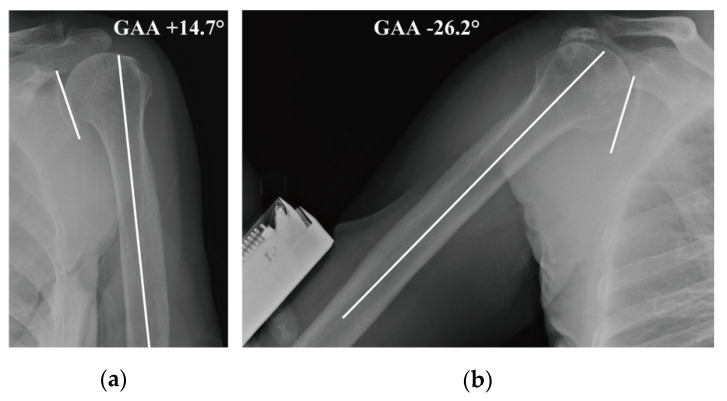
Radiographic glenohumeral adduction angle (a) Glenohumeral adduction angle (GAA) in a normal subject (b) GAA in a patient with rotator cuff tear and adduction restriction.
